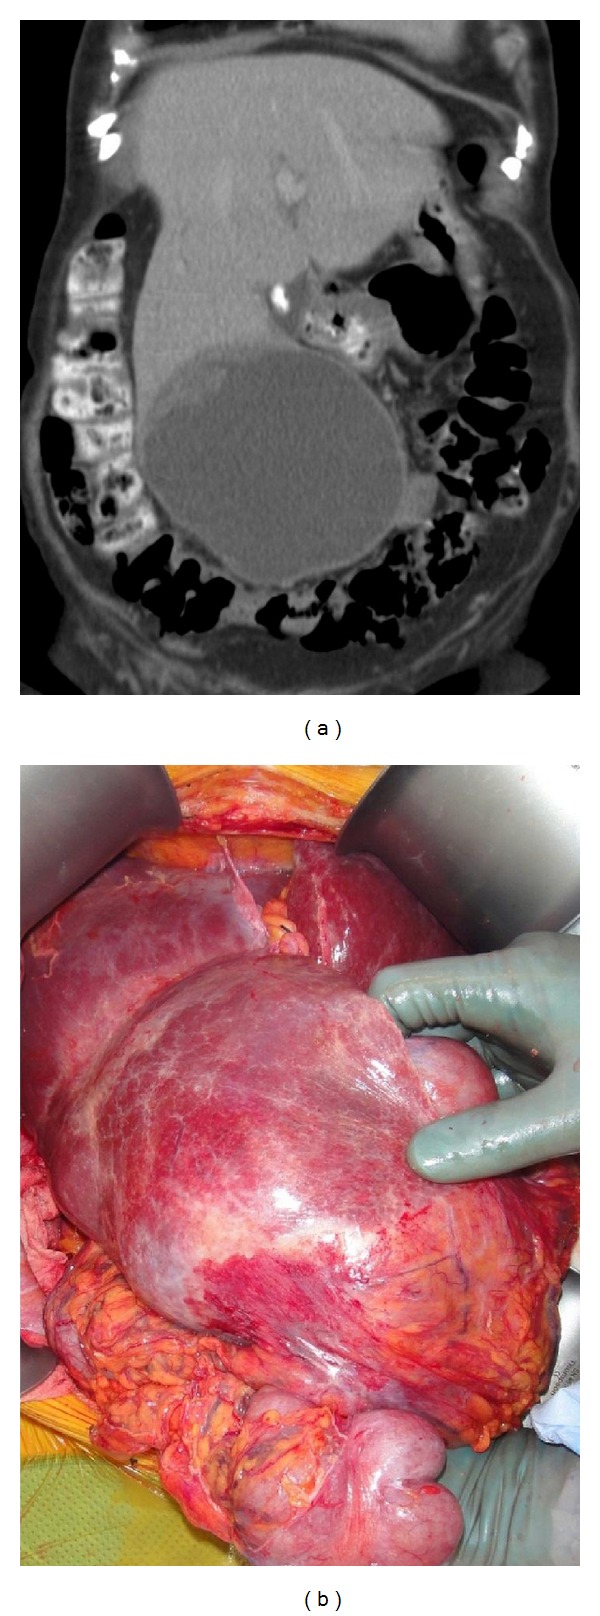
(a) Coronal computed tomography imaging demonstrating cystic tumour within the liver with more peripheral solid component. (b) Intraoperative image of large, partially haemorrhagic hepatic tumour overlying the right colon.
